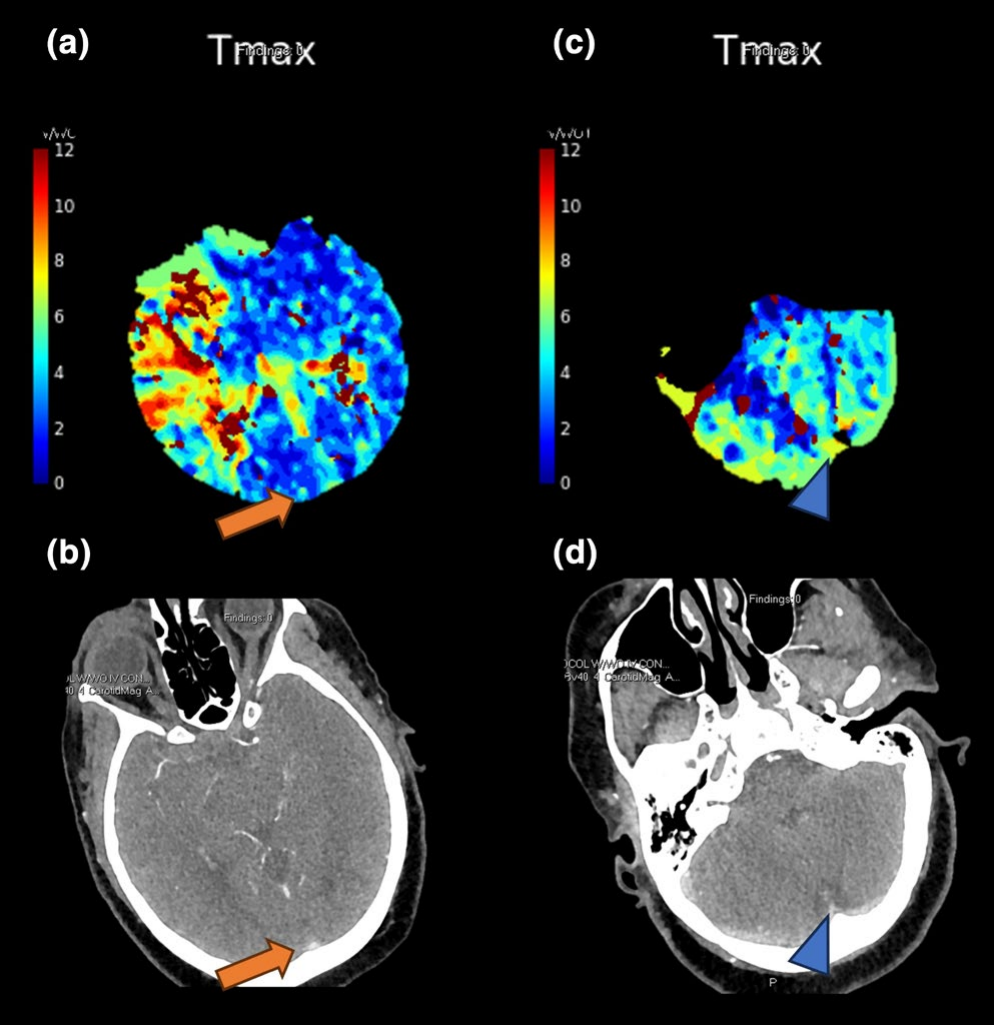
Imaging from a 72‐year‐old woman presenting with right proximal M1 occlusion and left extremity weakness. Her admission National Institutes of Health Stroke Scale score was 22. Post‐thrombectomy achieved modified Thrombolysis in Cerebral Infarction score 2c reperfusion after one pass. The 90‐day modified Rankin Scale score was 0. Top row: Tmax perfusion maps showing no prolonged venous transit in (a) the posterior superior sagittal sinus (orange arrow) or (c) the torcula (blue arrowhead). Bottom row: (b) and (d) corresponding computed tomography angiography images highlighting the venous structures (arrows).
